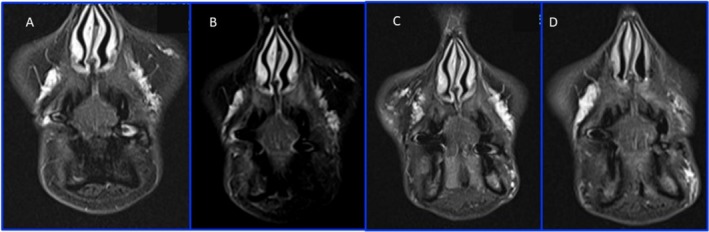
MRI: Coronal T2 TIRM in I (A), II (B), e III (C and D) MRI, showing the persistence of HA filler in the pyriform recess 24 months after the first treatment.
